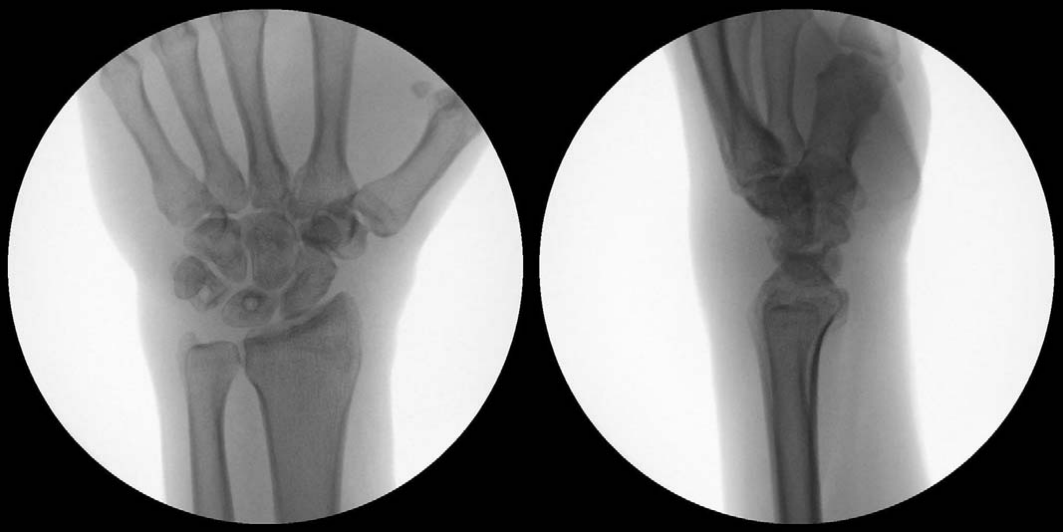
Posteroanterior and lateral radiographs at final follow-up.
